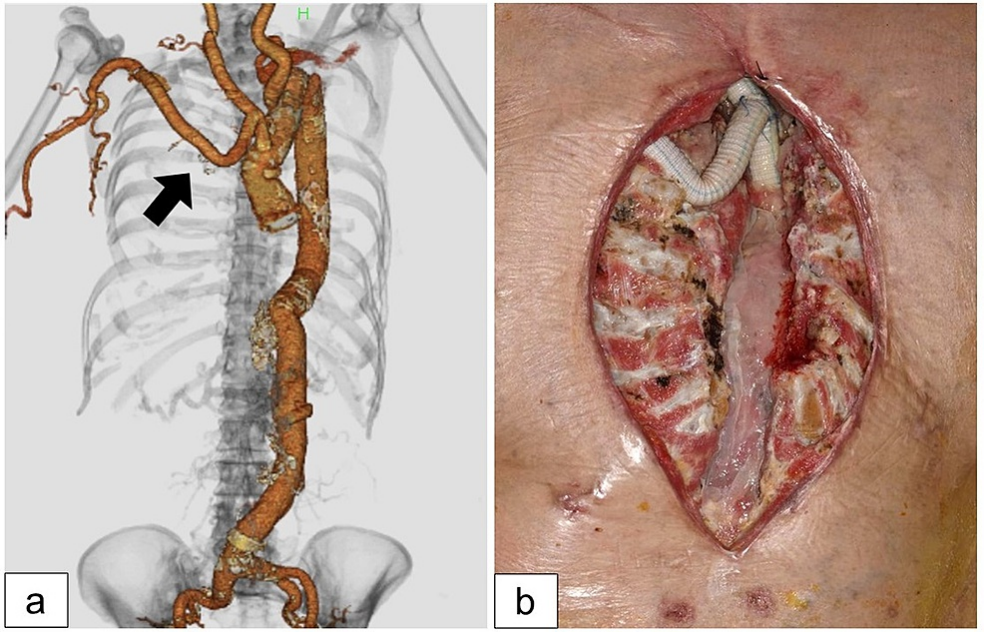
On admission. (a) Computed tomography angiography of the aorta. The arrow shows the replaced prosthetic graft during the last operation. (b) The infected prosthetic graft was exposed within the mediastinal wound without the sternal bone.
